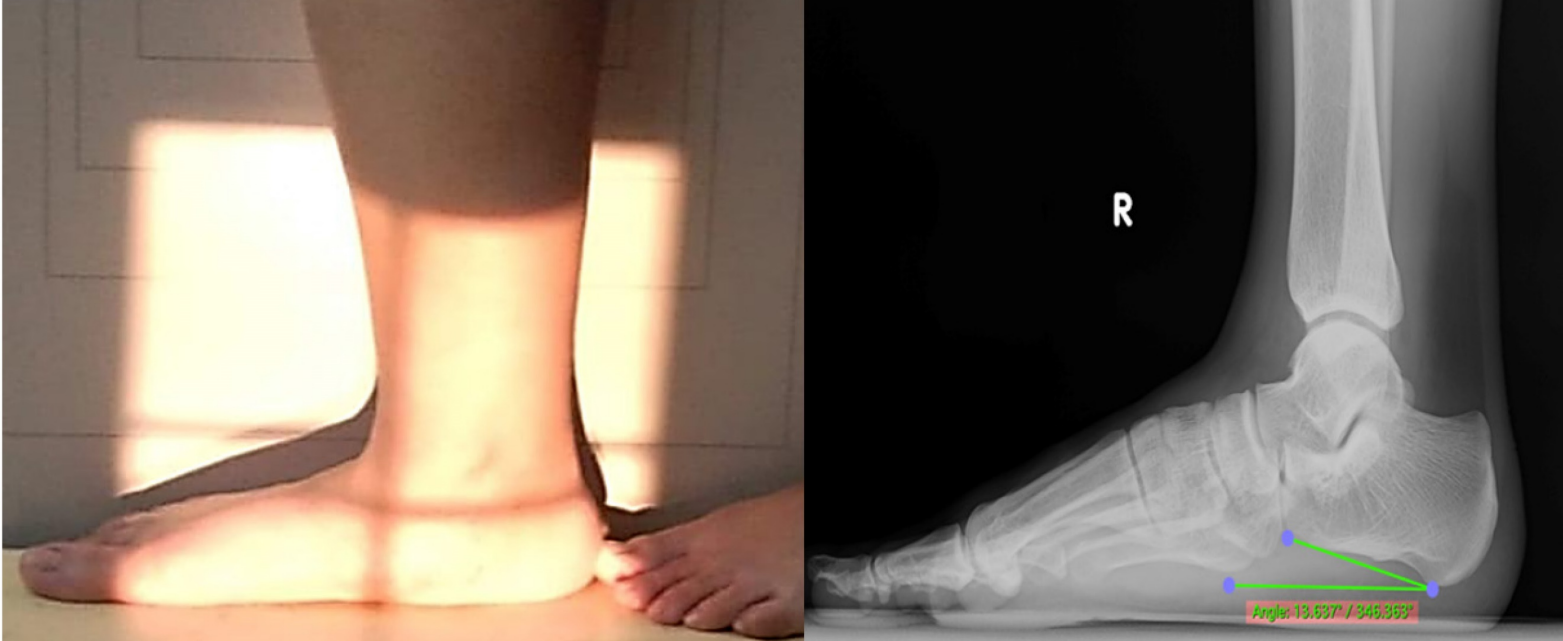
Positioning of lateral x-ray weight bearing foot.
